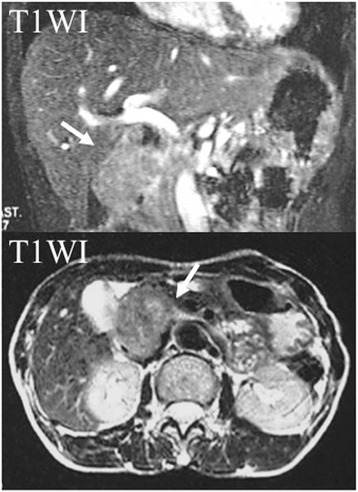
Abdominal magnetic resonance imaging. The *arrow* indicates the tumor. Magnetic resonance imaging shows a well-defined lesion along the course of the IVC. *IVC* inferior vena cava
